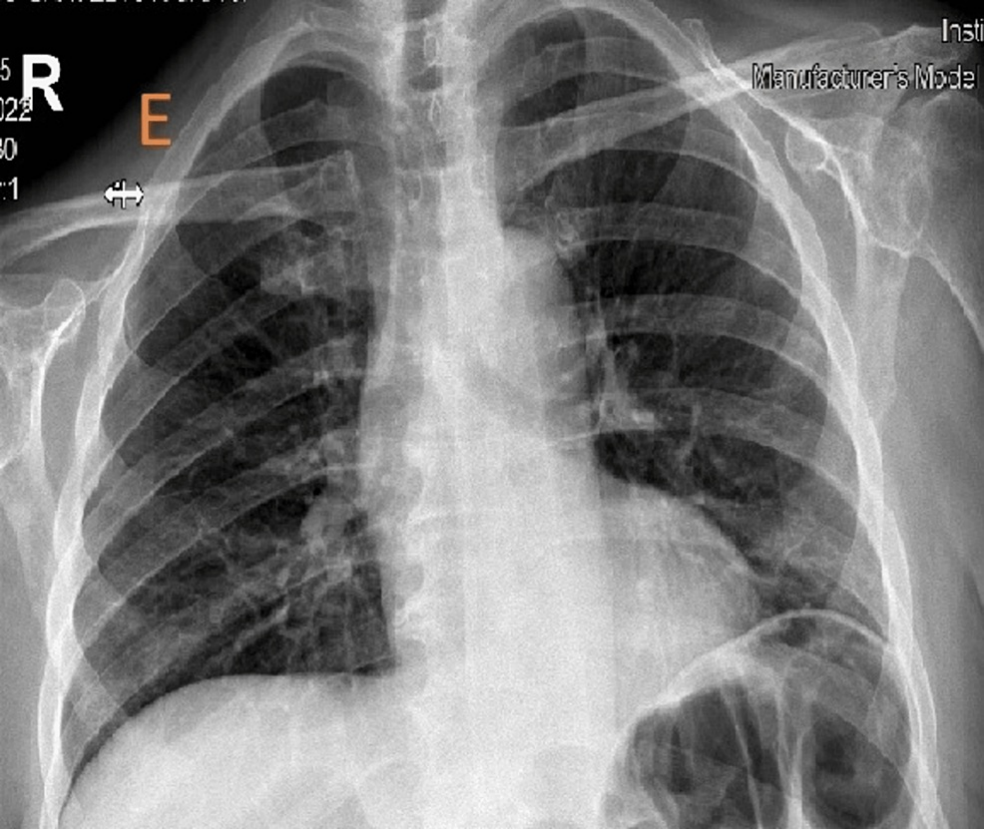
Upright Chest X-ray after two months. Complete resolution of the pneumoperitoneum.
